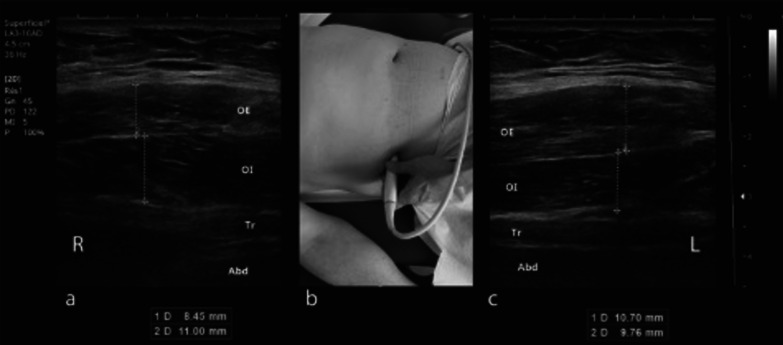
Ultrasound of the right flank (a), with thickness measurement of the external oblique (EO) at 8.45 mm and of the internal oblique (IO) at 11 mm. Ultrasound was performed bilaterally placing the probe at shown location (b) comparatively on both sides, finding at left (c) an EO at 10.7 mm and IO at 9.76 mm. We noticed a difference of 1 mm on the left side and 2.55 mm on the right side (and an inversion of the relative thickness)
